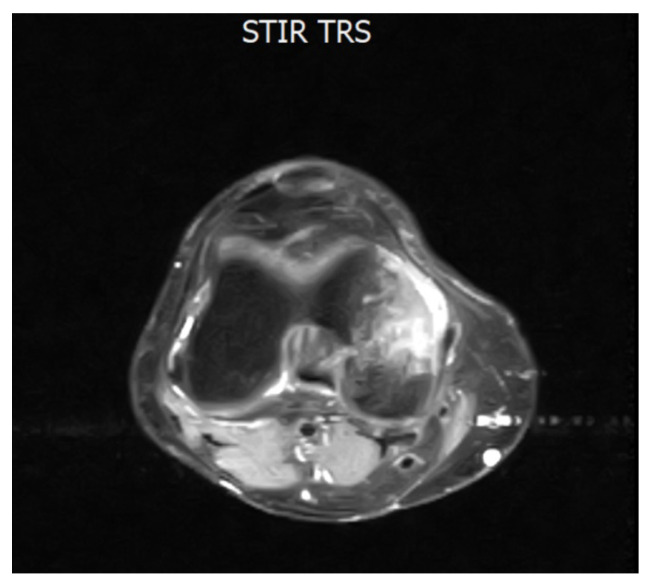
Axial MRI section of the knee showing intra-spongious bone marrow edema of the medial femoral condyle. The hyperintense signal is visible on STIR and T2 fat-saturated sequences, consistent with marrow edema. This image dates to February 2021, when the patient completed 28 months of TKI treatment with DAS and referred to a sudden onset of joint pain. Abbreviations: STIR (short tau inversion recovery) and TRS (tool rotation speed).
